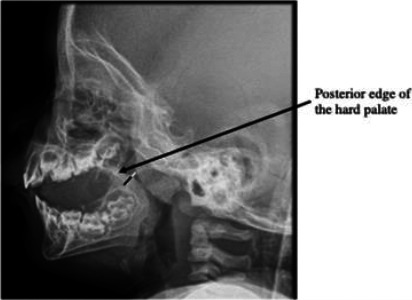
Lateral radiograph of the postnasal space showing Cohen's method for assessing adenoidal enlargement. The thickness of the soft palate (thick black line) is measured 1cm away (0.5cm in children < 3 years of age) from the posterior edge of the hard palate (black arrow). The width of the adjacent nasopharyngeal airway (short white line) is also measured. In this child, the airway is narrower than half the thickness of the soft palate; the adenoid (lobulated soft tissue density at the posterior pharyngeal wall) was therefore reported as severely enlarged
